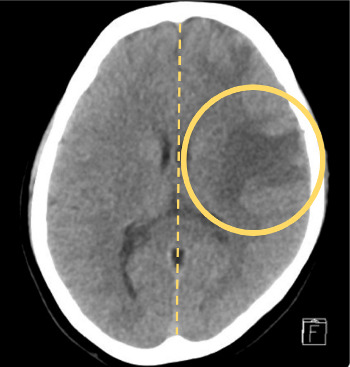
CT head: large hypodensity of the left frontoparietotemporal cerebral hemisphere (yellow circle). There is an associated mild, less than 5 mm, midline shift noted toward the right side (dashed yellow line).
